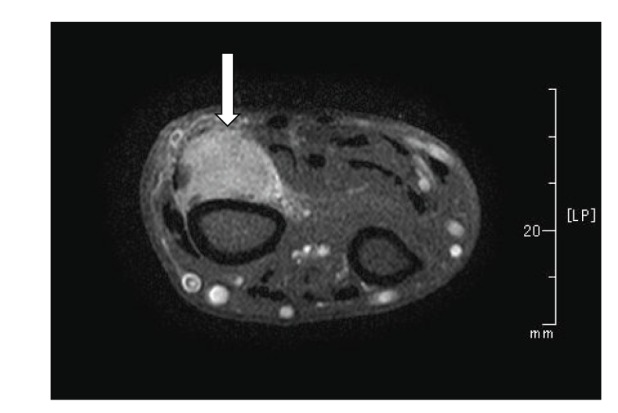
Gadolinium enhanced MRI image of the patient's right wrist.White arrow indicates the tumor.
